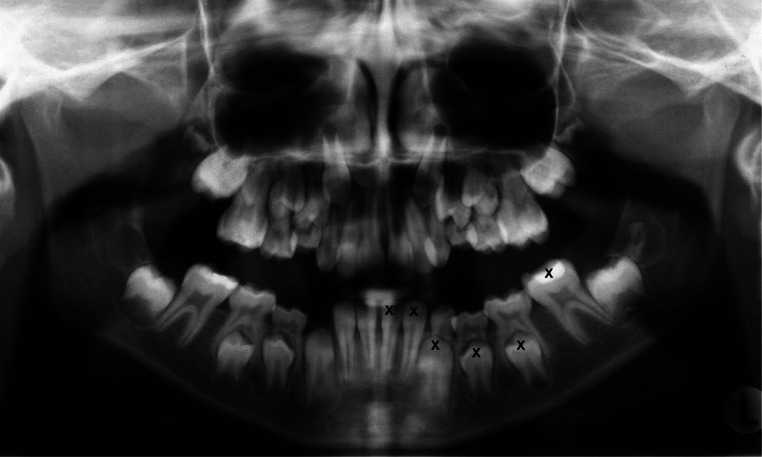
Panoramic radiograph from one of the included individuals. Teeth 31–37, which are marked with a cross, were assessed according to Demirjian et al. [15]. The criteria for the assessment of different stages varied from the beginning of calcification of crown to apical root apex completely closed (stages A–H). In Fig. 1, for example, tooth 31 was assessed as stage H and tooth 37 as stage D. If a tooth appeared to be between stages, the earlier stage was chosen
